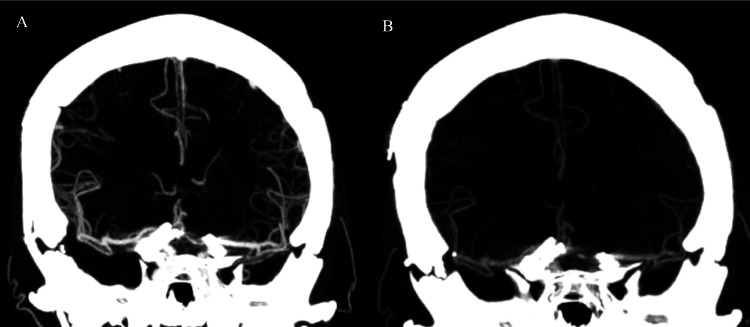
Computed tomographic angiographies after the second microsurgical treatment In the angiographies performed at one (A) and three years (B) after the second clipping, there was no evidence of residual filling in the recurrent A1-A2 aneurysm.
